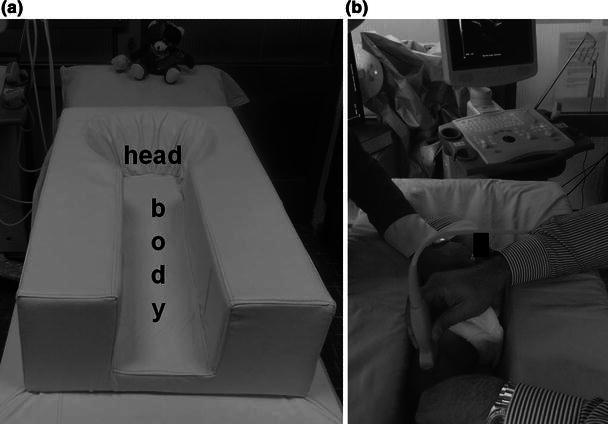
a The special positioning apparatus that maintains the baby in the lateral decubitus position. b Ultrasonographic examination of the right hip of a baby in the special positioning apparatus
